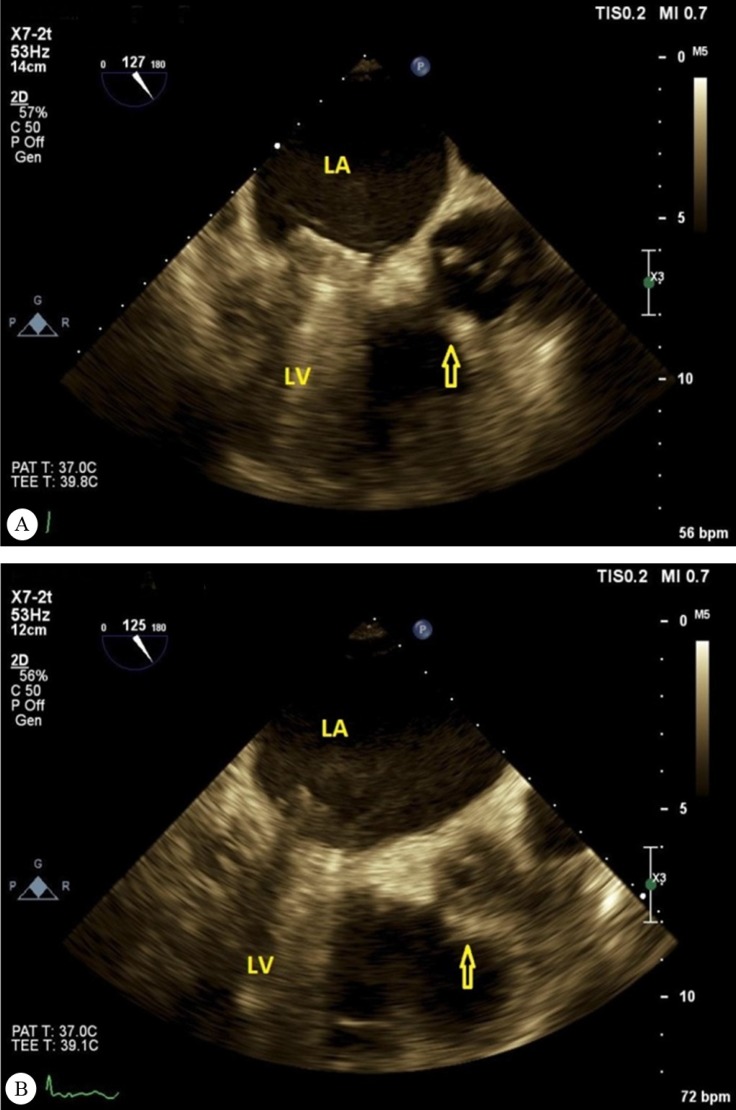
A) Pseudoaneurysm (arrow) of the ascending aorta in the second transesophageal echocardiography (long-axis view), depicted after the resolution of the thrombosis. B) The same pseudoaneurysm (arrow) of the ascending aorta in the same view in the primary transesophageal echocardiography, presenting a challenge in diagnosis because it was filled with a thrombosis.
